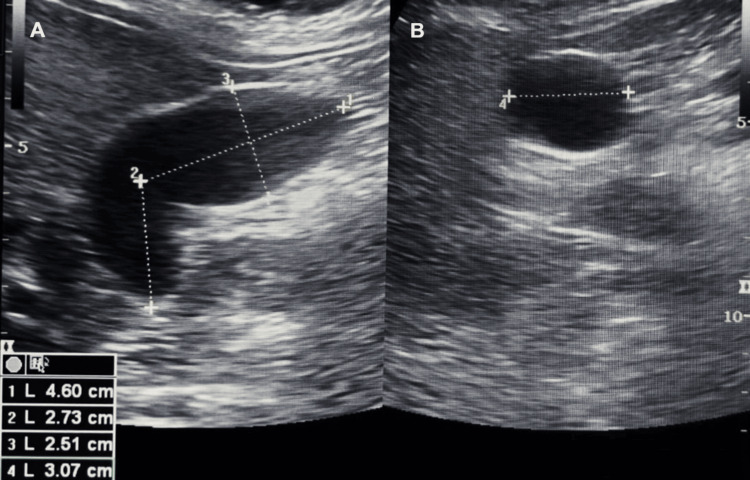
Follow-up abdominal ultrasound Images A) and B) confirming a reduction in gallbladder volume (29 cc) and showing no compression of the common bile duct.
